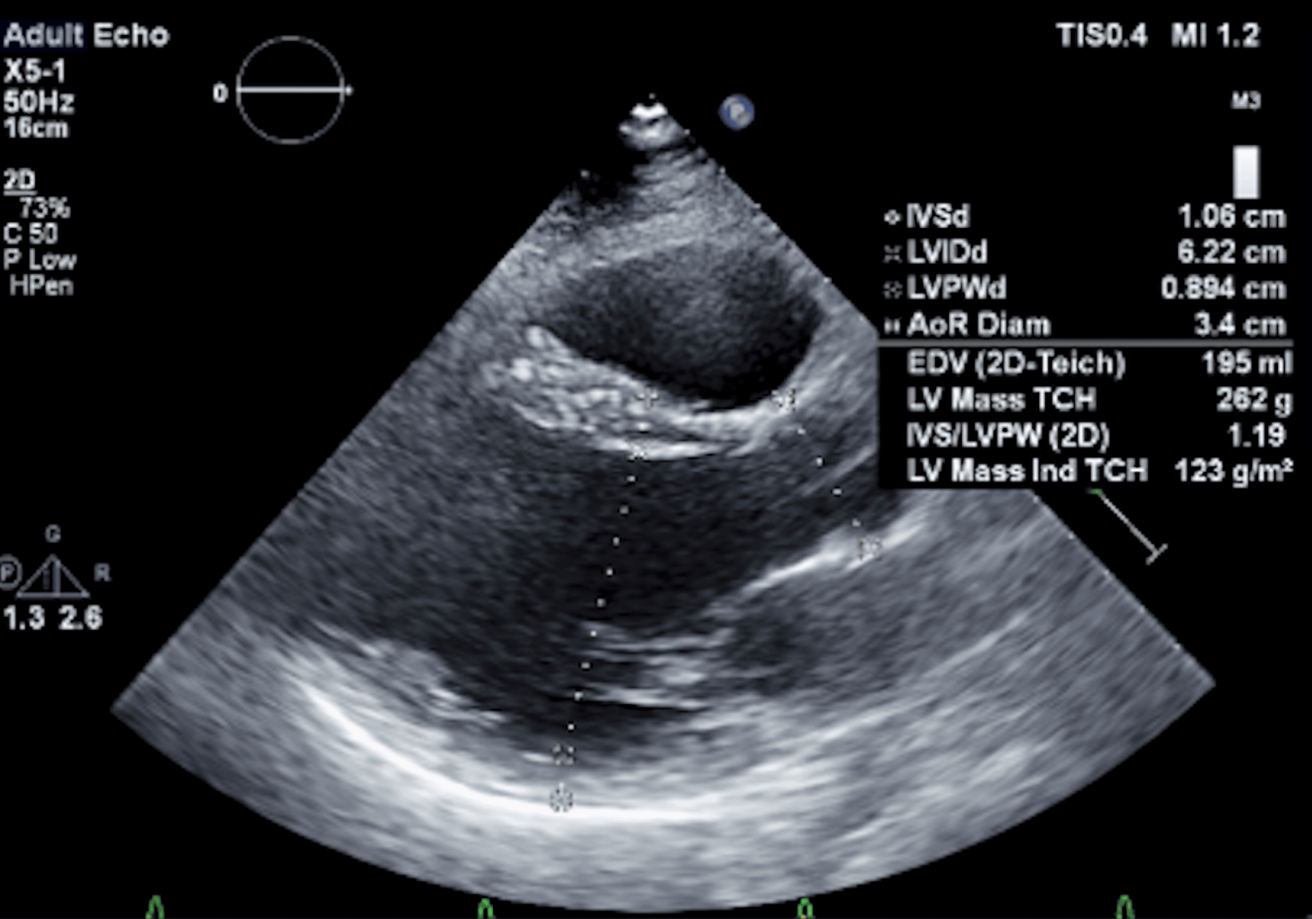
Parasternal long-axis TTE demonstrated eccentric hypertrophy (increased left ventricular mass index and normal relative wall thickness). Dynamic heart 3D modeling of the left ventricle revealed an ejection fraction of 38%. TTE: transthoracic echocardiography
